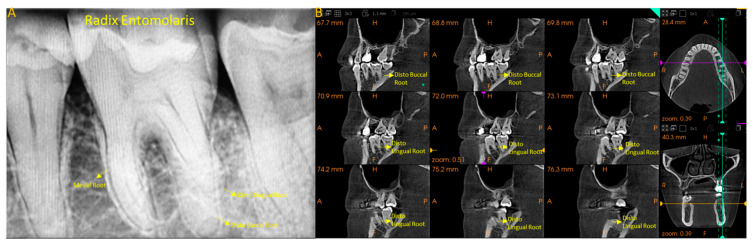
Radix Entomolaris. (A), intraoral periapical radiograph of MFM showing three roots: mesial, distobuccal, and distolingual (DLR); (B), CBCT image of the same showing the presence of a separate DLR.
